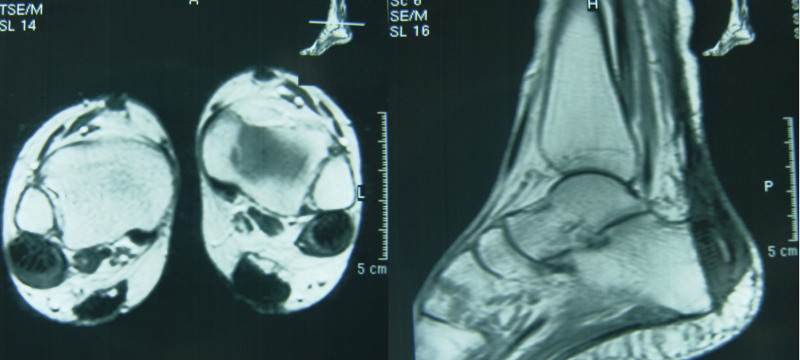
Magnetic resonance imaging (MRI) scan of the reconstructed Achilles tendon postoperative 2.5 yr. Both axial and saggital magnetic resonance image of the reconstructed Achilles tendonshowing a tendon-like structure.
